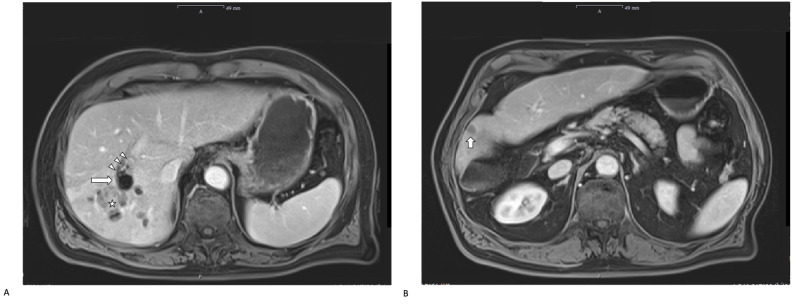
Preoperative imaging Preoperative MRI was performed a week prior to hepatectomy and shows a considerable degree of hypertrophy of the left liver lobe following portal vein embolization. The MRI demonstrates the hepatic lesions in segment V (indicated by the white star, A), and in segment VII (indicated by the white short arrow, B). The white long arrow in 1A indicates microspheres in the right portal vein with adherent thrombotic material (indicated by three white triangles).
